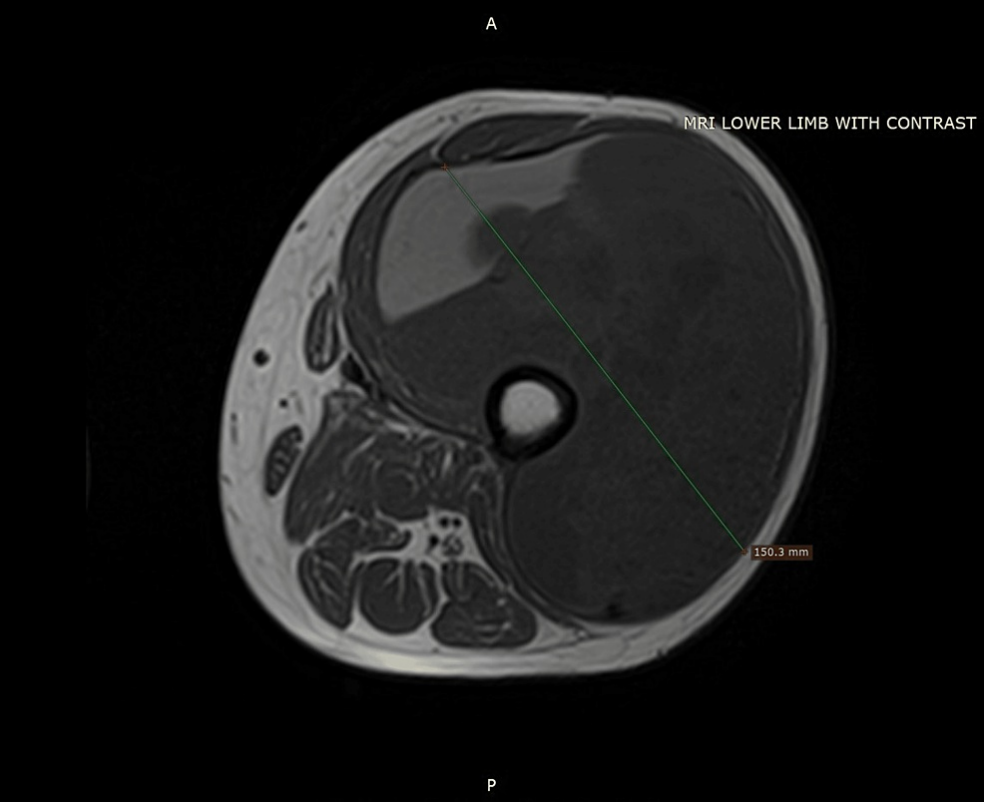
Shows the T1 MRI axial dimensions of the tumor involving anterolateral compartment of thigh.
